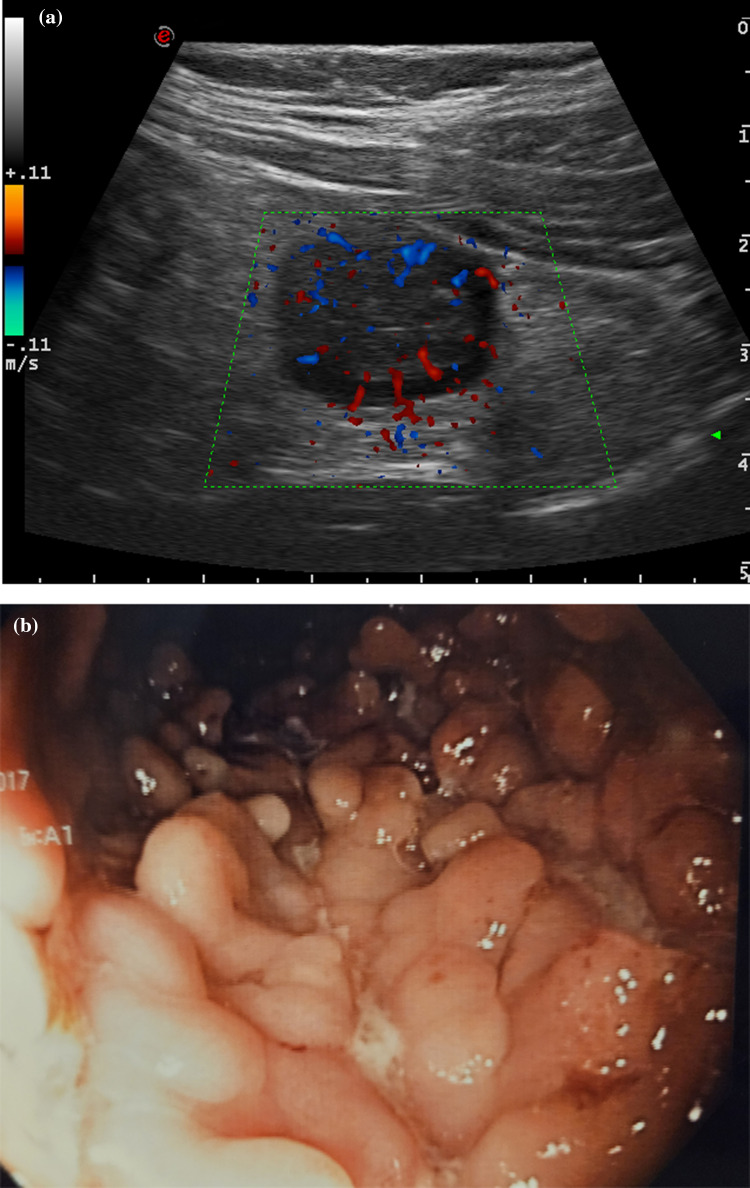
Cross sectional intestinal ultrasound still image (a) of the sigmoid in a patients with colonic Crohn’s disease in clinical remission showing massive pseudopolyposis as an increased anteroposterior diameter with color Doppler signals in the wall spreading within the lumen. Endoscopic features of the correspondent sigmoid colon, showing massive pseudopolyposis (b)
